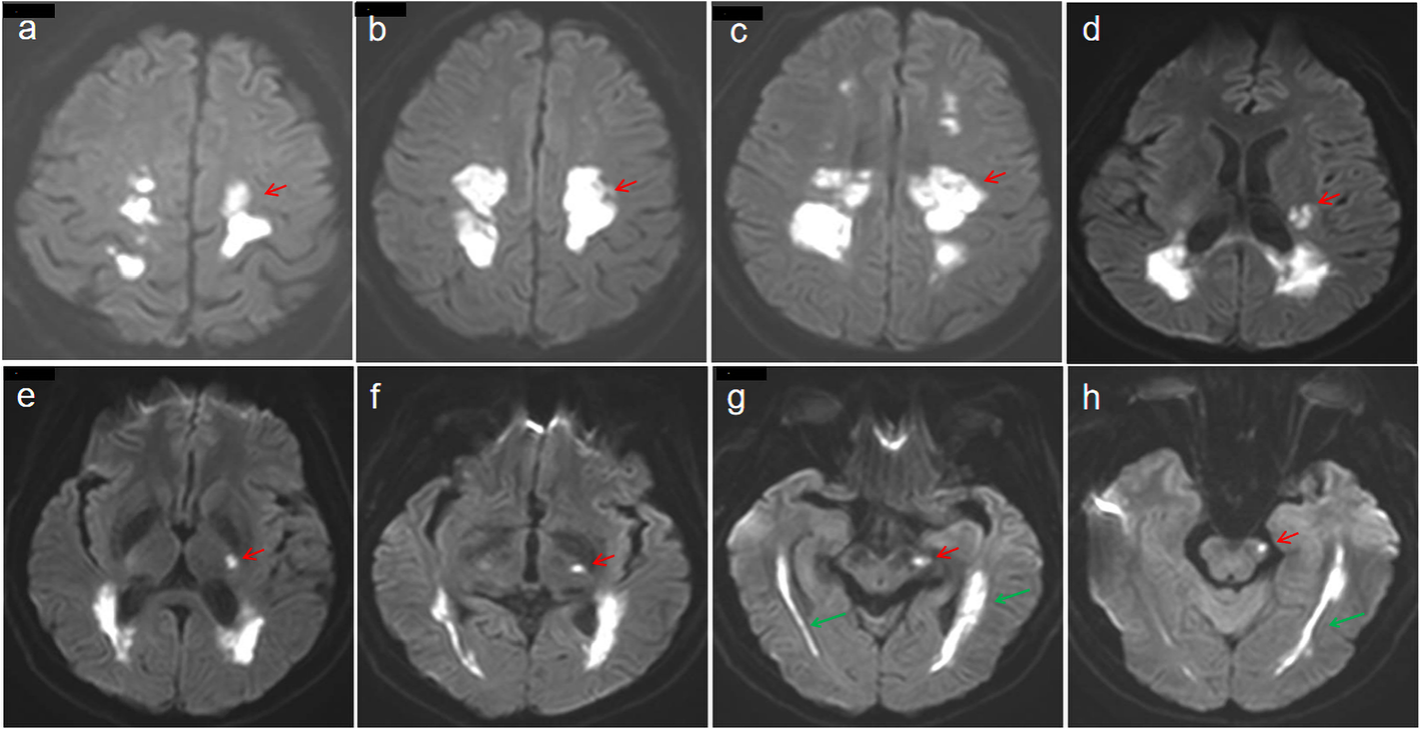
DWI sequences on different levels of the brain in April 2020. The bilateral pyramidal tracts had high signal intensity at different levels (a-h), especially on the left side (g, h). The diffusion limitation of the bilateral optic radiation (green arrows) and the corticobulbar tract (red arrows) extends from the cerebral cortex to the midbrain
